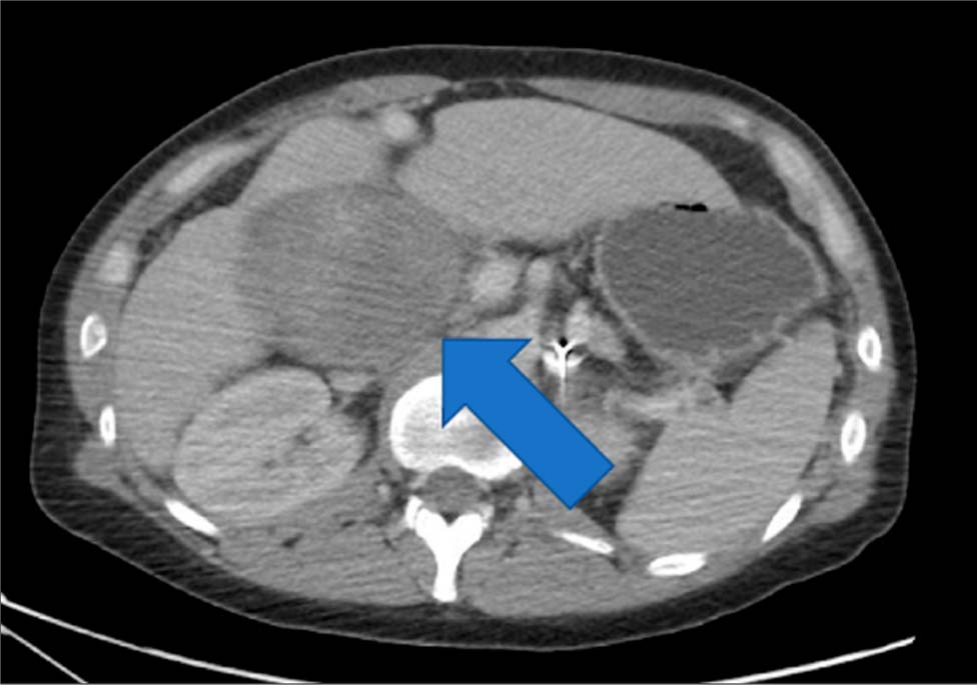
Computed tomography (CT) with intravenous contrast of the abdomen in the axial view showing biliary sludge and gallbladder distension with surrounding edematous changes at the blue arrow consistent with acute cholecystitis.
